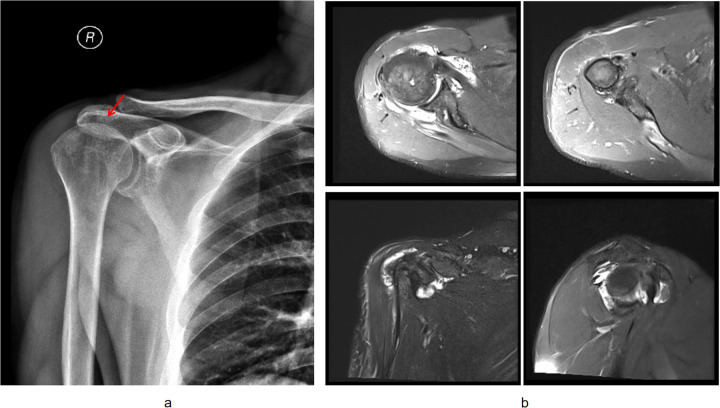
(a) anteroposterior radiograph of the right shoulder demonstrating cortical irregularity and subtle bone density changes involving the undersurface of the acromion (red arrow), suggestive of focal bone erosion; (b) coronal T2-weighted fat-suppressed MRI showing extensive hypointense synovial proliferation within the glenohumeral joint and subacromial space, consistent with PVNS, along with a full-thickness supraspinatus tendon tear.
